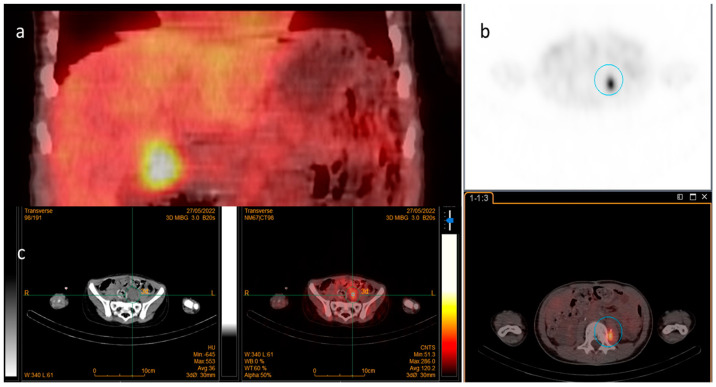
Iodine-123-MIBG SPECT CT images in three different patients with local uptake: (a) hepatic hilum; (b) left psoas muscle; (c) pelvic.
